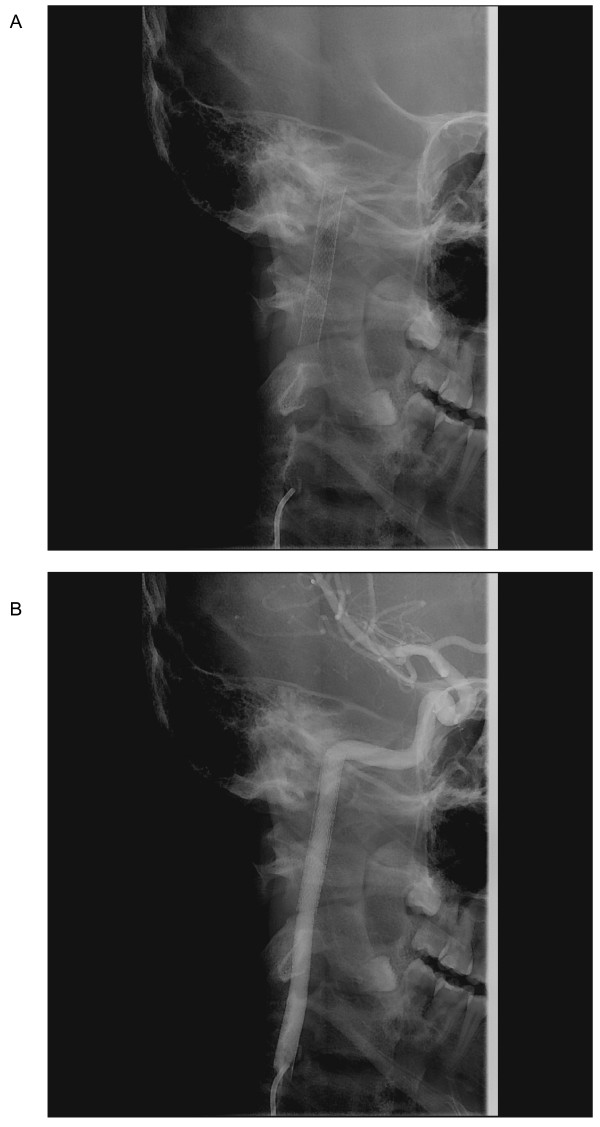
(A) Oblique neck xray shows stent deployment, and (B) follow-up catheter angiography at 6-months poststenting with right cervical ICA injection shows restoration of the normal carotid lumen without vessel wall.
